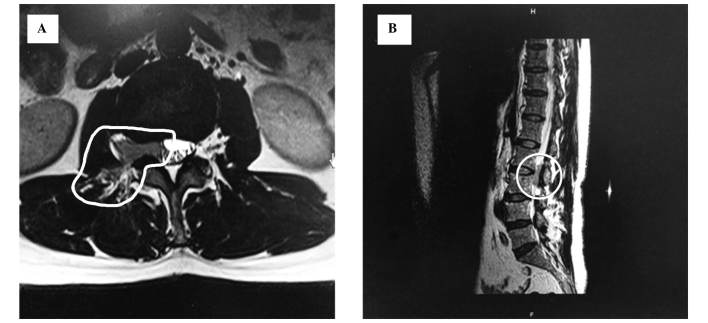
Contrast enhanced magnetic resonance imaging of the lumbar spine revealed (A) a 4.9×2.1×1.8 cm homogeneously enhanced and (B) ill-defined mass from vertebrae L2–L3.
